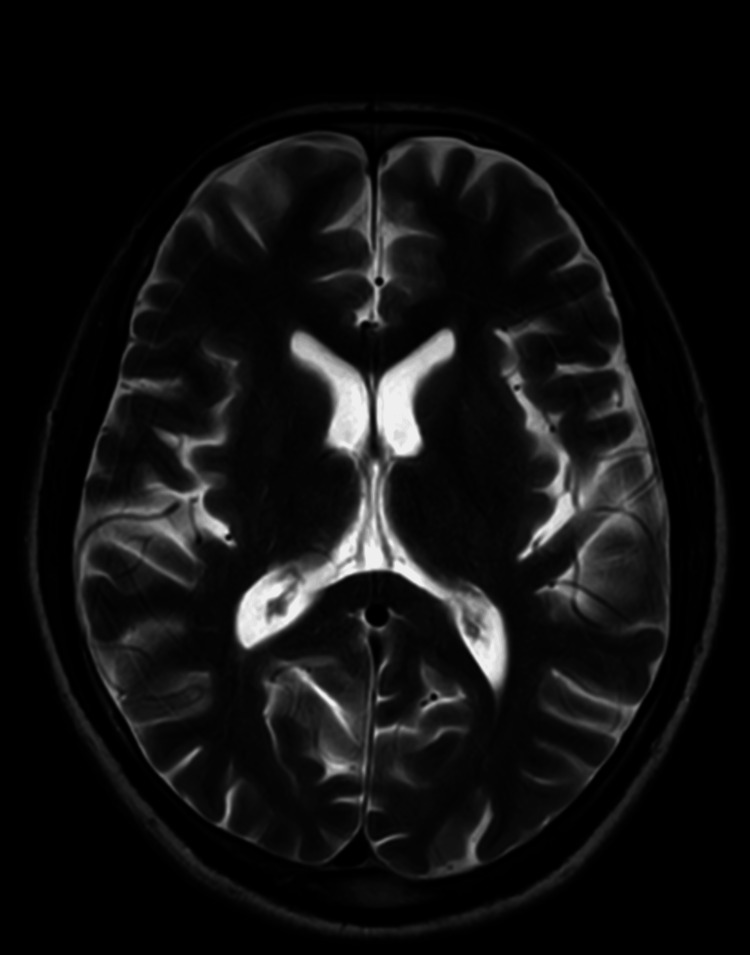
MRI brain plain suggestive of no structural abnormalities or lesions affecting the facial nerve
